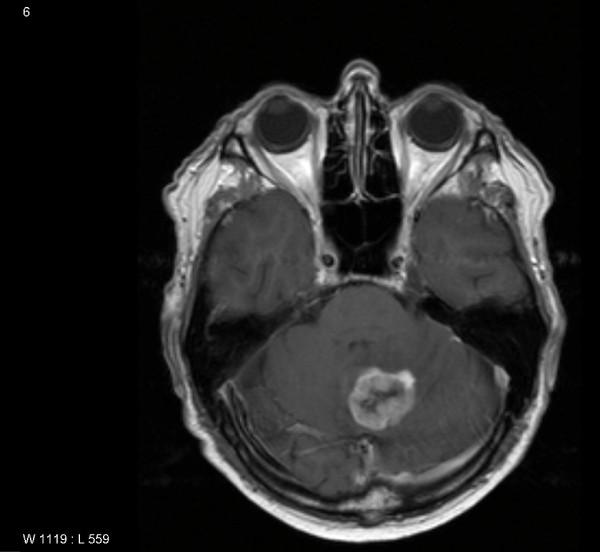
Preoperative T1-weighted magnetic resonance imaging showing a 3 cm large contrast-enhancing infratentorial tumor.
